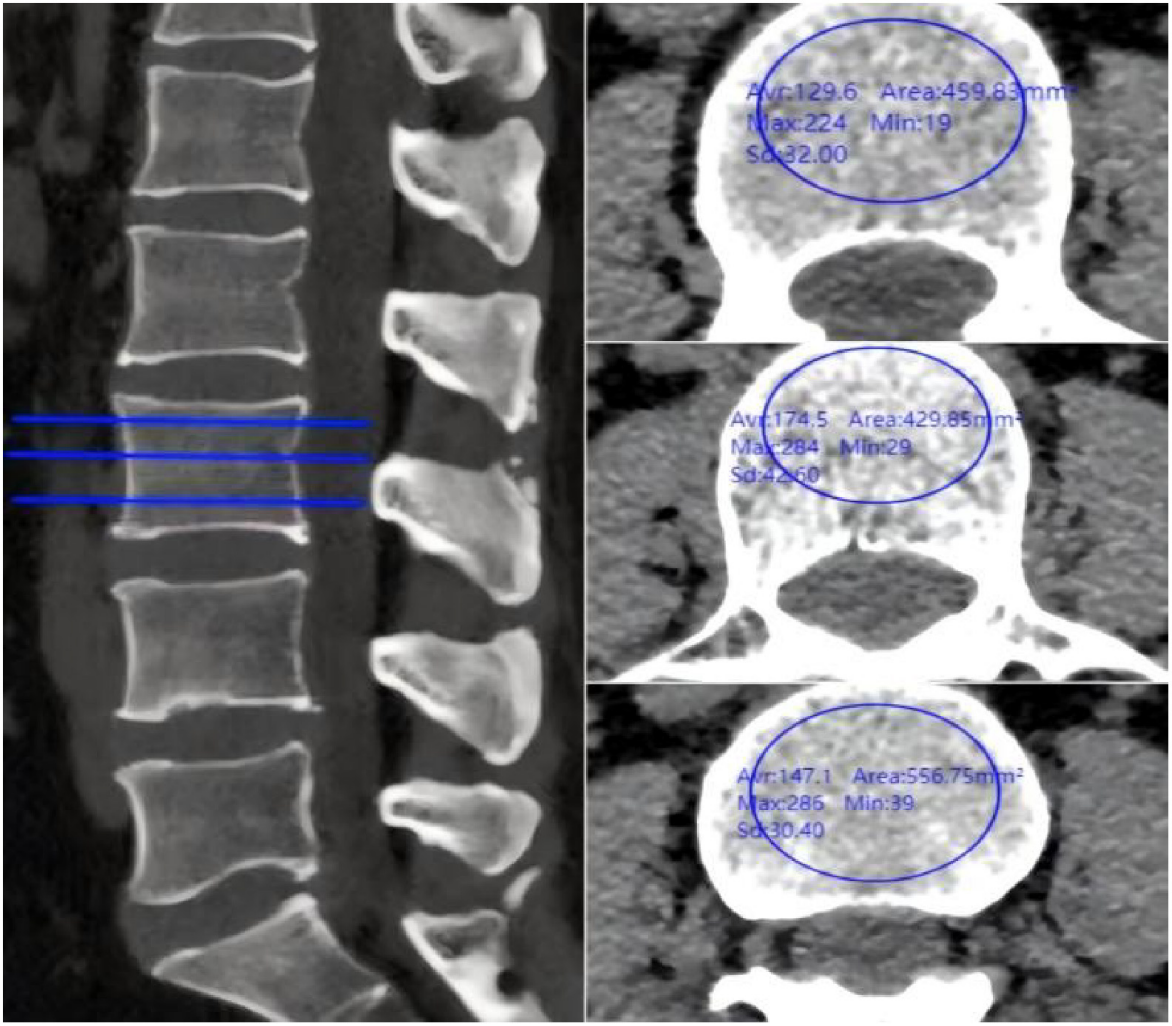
Preoperative lumbar CT showing individual vertebral HU measurements.
